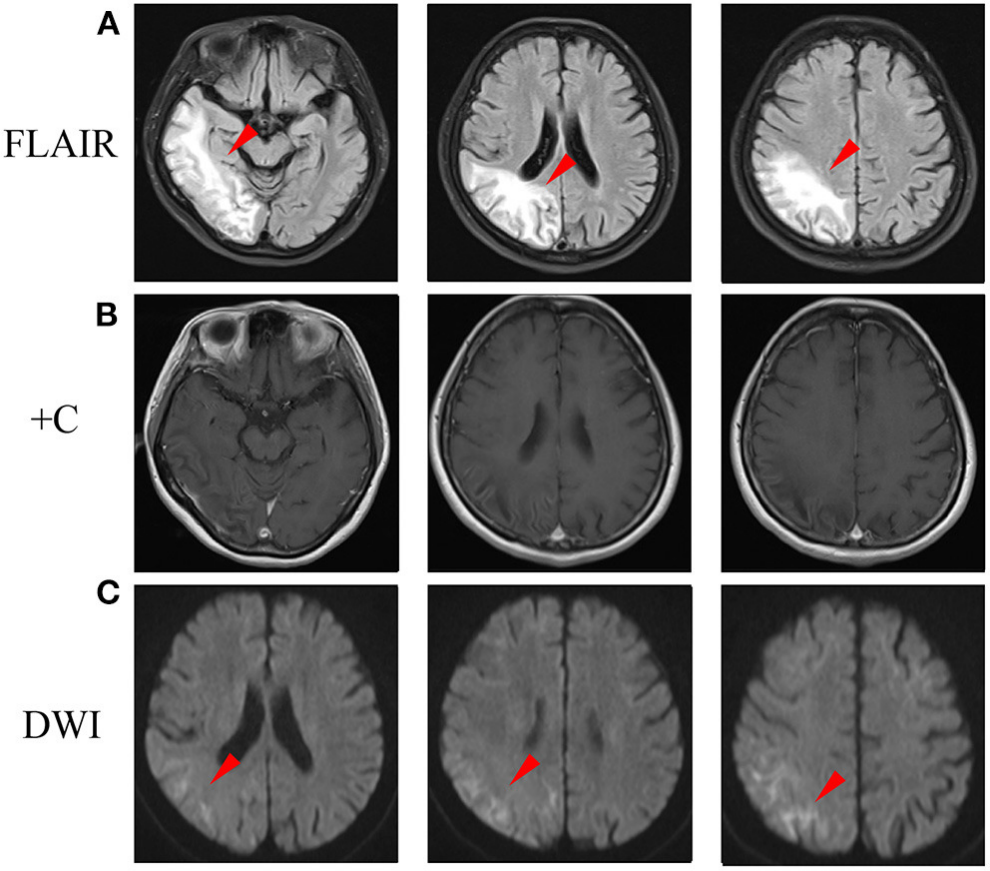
A follow-up brain MRI performed 3 months later after disease onset. (A) FLAIR images showed abnormal hyperintensity was improved in cortex, while progressed in the subcortical area (arrowheads). (B) Post-contrast MRI showed cortical enhancement was reduced. (C) DWI showed hyperintensity in the corticomedullary junction progressed (arrowheads). DWI, diffusion-weighted image; FLAIR, fluid-attenuated inversion recovery.
